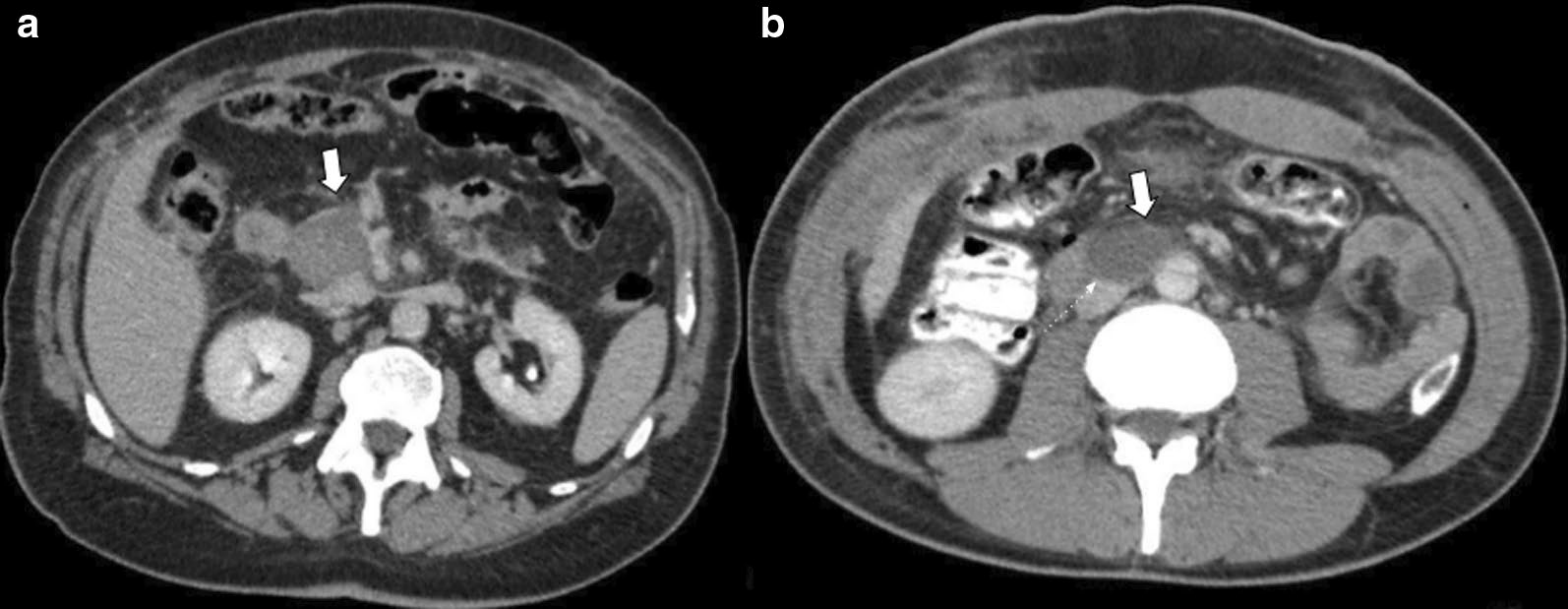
Pancreatic fistula vs. Abscess. Two different patients. Axial portal venous phase CT scans: (a) 73-year-old male patient with abdominal pain 25 days after pancreaticoduodenectomy due to pancreatic neuroendocrine tumor. (b) 45-year-old male patient with fever 21 days after pancreaticoduodenectomy due to ampulloma. Both present an homogeneous fluid collection near the PJ (big arrow). In patient B impresses to exist a thin wall during portal phase (dotted arrow). Both of them were percutaneous drainage with elevated amylase concentration in A and purulent fluid in B.
